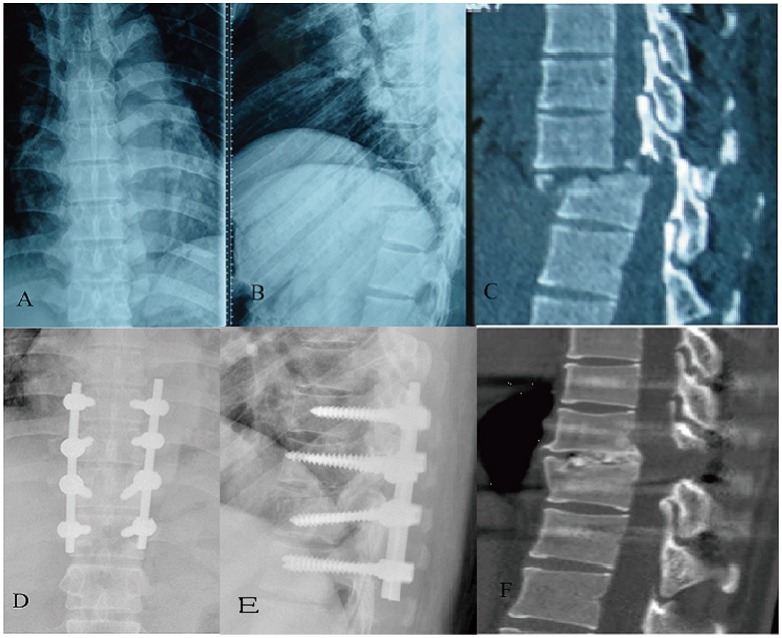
The anteroposterior and lateral X-ray films (A and B) of a 34-year-old man sent to our hospital after an accidental fall show a T9–T10 fracture and dislocation, and the injury was confirmed by CT reconstruction (C). The patient's ASIA sensory score was 173, and his motor score was 67. We performed transforaminal decompression and interbody fusion on him. The anteroposterior and lateral X-ray films (D and E) at 3 months postoperation show the internal instruments placed in a good position. Intervertebral bony fusion between T9–T10 was observed on the CT images at 36 months postoperation (F). The patient's ASIA sensory score was 216, and his motor score was 96.
